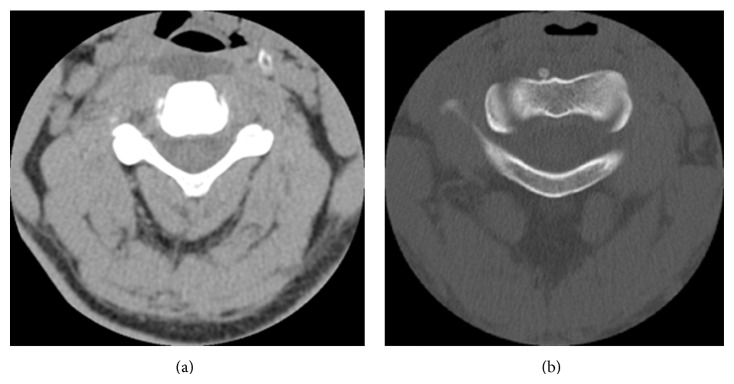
Axial noncontrast CT scan of the cervical spine demonstrating a retropharyngeal fluid collection at the level of C4 (a) and a calcification within the longus colli muscle anterior to C2 (b).
